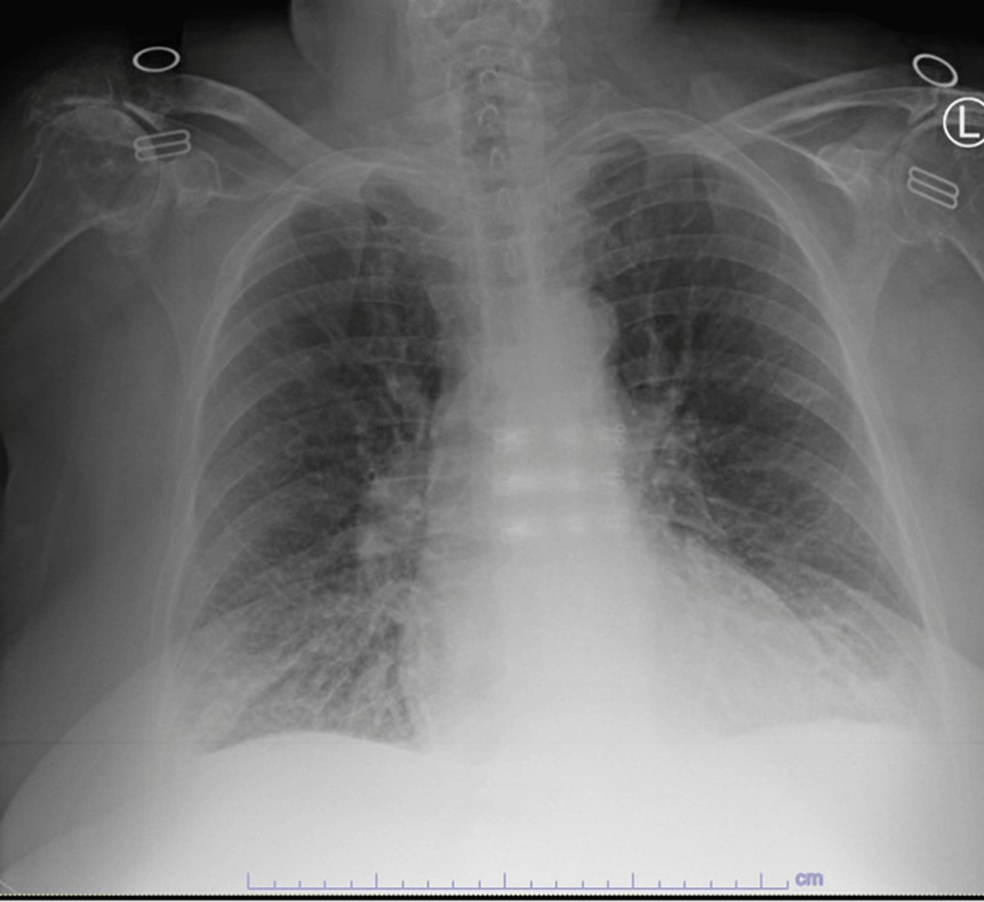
Chest x-ray showing mild patchy increased opacities in the lung bases possibly representing mild edema or atypical pneumonia in the appropriate clinical setting.
